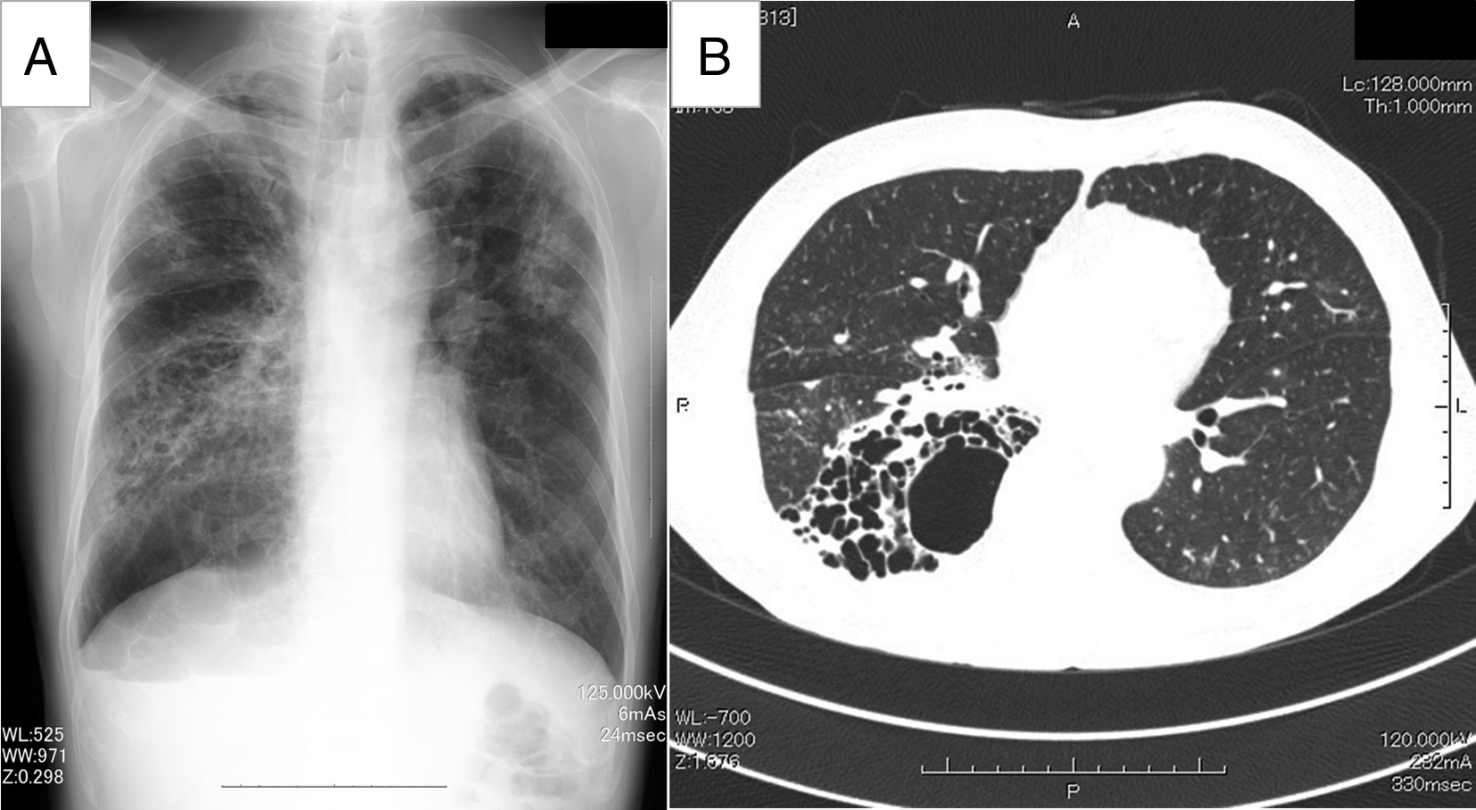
Case 1: (A) chest X‐ray image at admission showed infiltrative shadows in the left upper and right lower lung fields. (B) Chest computed tomography (CT) scan at admission showed multilocular cystic shadows together with air fluid levels in the right lower lobe and patchy shadows and cord‐like shadows in both the upper lung lobes.
